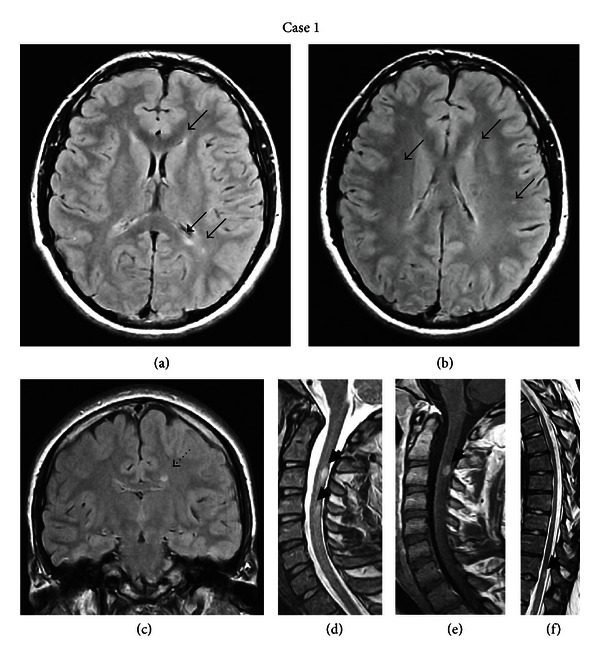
(a) and (b): Axial FLAIR brain MRI of the first patient demonstrating right subcortical and juxtacortical and left periventricular hyperintense lesions (black arrows). (c) Coronal FLAIR brain MRI showing a left periventricular hyperintense lesion (dotted black arrow). (d) Sagittal T2-weighted MRI of the cervical spine showing hyperintense lesions on C3 and C4-C5 level (black arrowheads). (e) The lesion on C3 level appears to be enhanced on T1-weighted image (black arrowhead). (f) Sagittal T2-weighted MRI of the thorasic spine demonstrating a hyperintense lesion on T11 level (black arrowhead).
